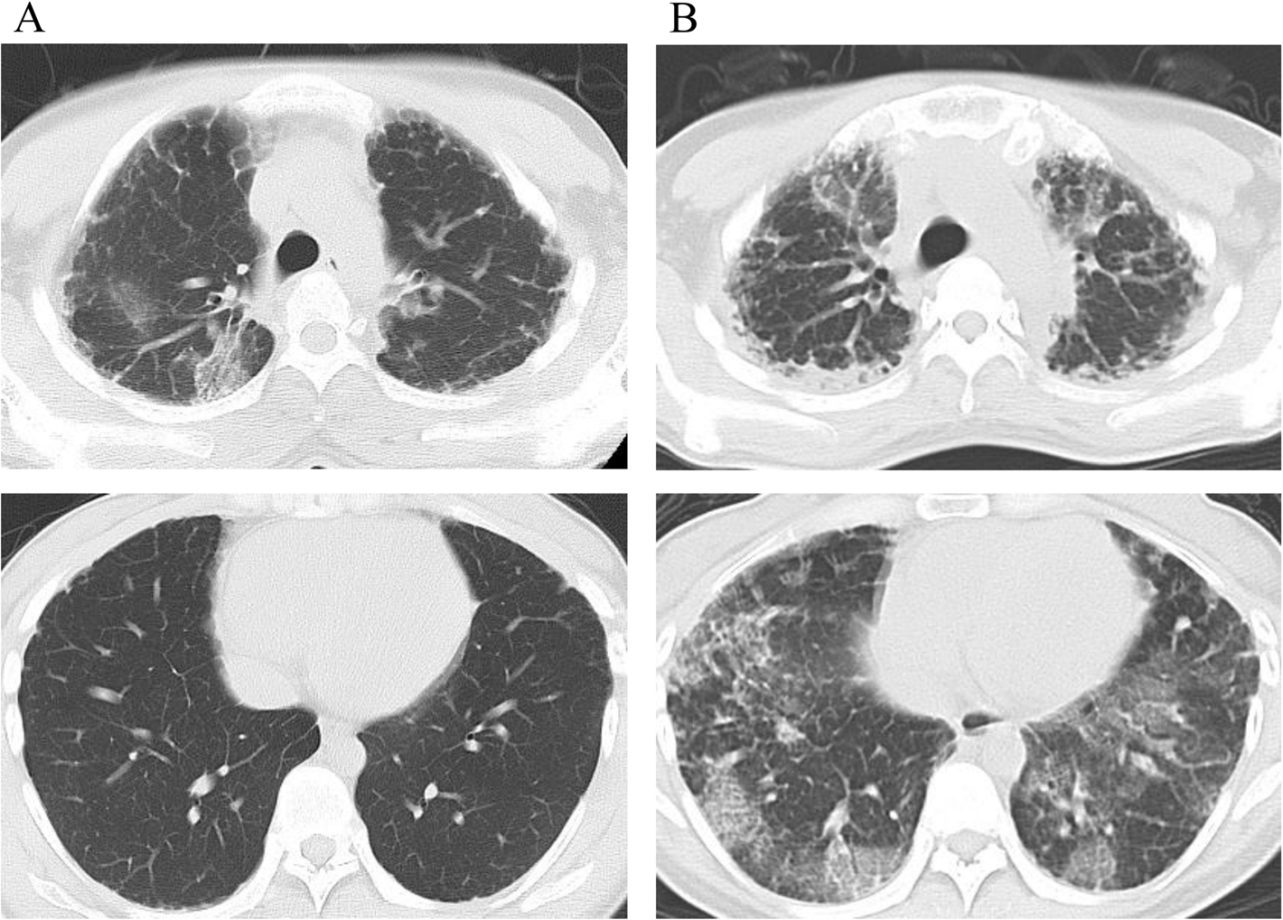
Chest computed tomography findings (CT). (a) Chest CT 3 years before admission showed apical pleural thickening and reticular opacity in the peripheral lung field (b) Chest CT at 24 weeks of gestation showed upper lobe-predominant pleural thickening, bronchiectasis, reticular opacity, and ground-glass opacity throughout the lung
